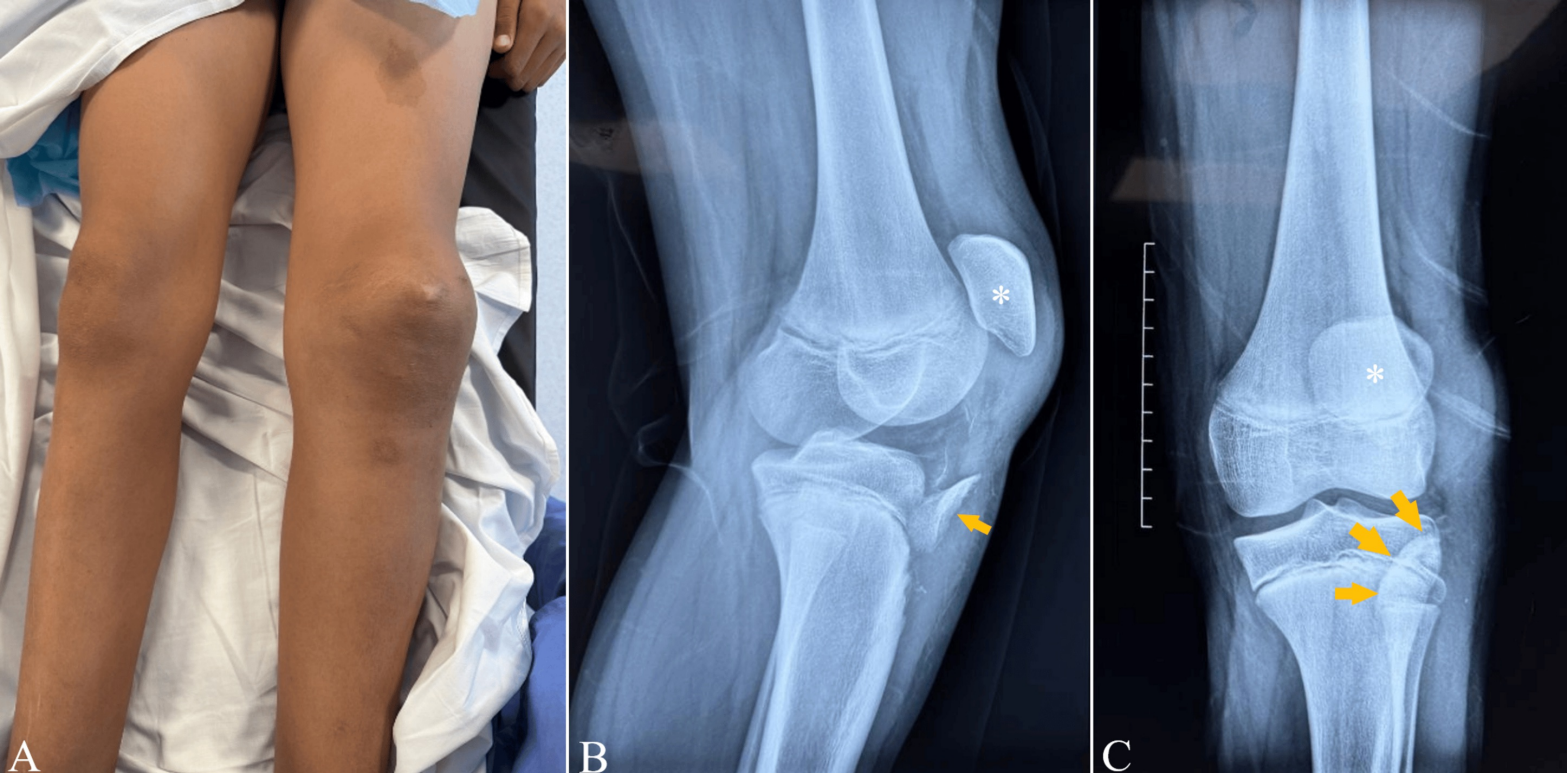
Clinical appearance of the left knee at admission (A). Preoperative X-rays of the left knee showing an avulsion fracture of the tibial tubercle (arrows) with patella alta (asterisk) on the lateral (B) and anteroposterior (C) views
